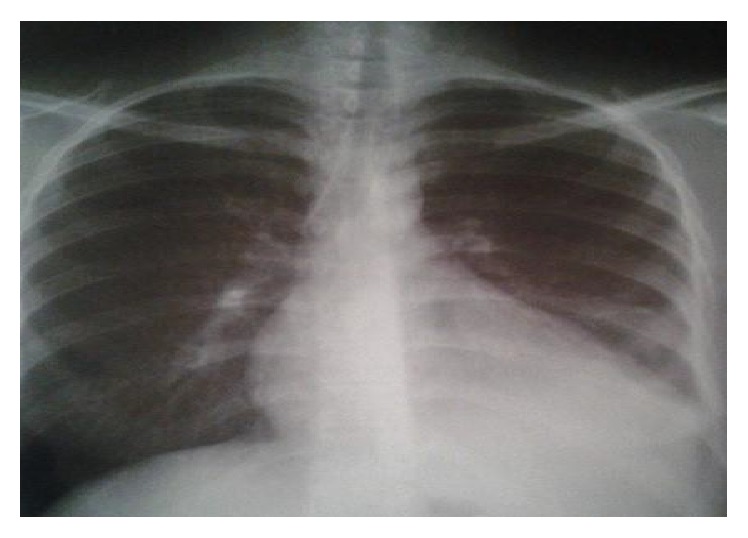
Control of chest X-ray after fluid evacuation.
